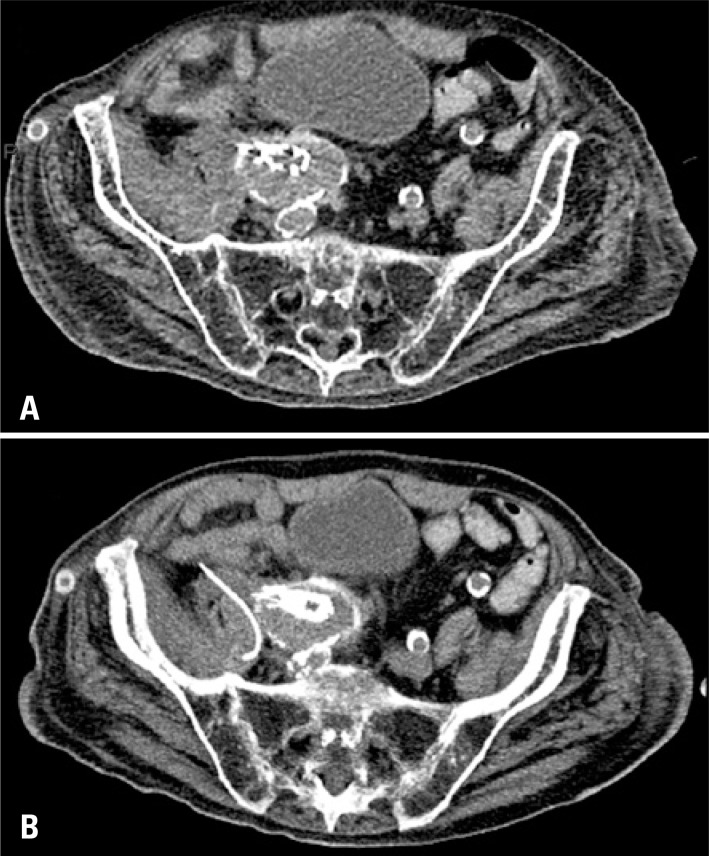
Percutaneous treatment of endograft-related psoas abscess. (A) Non-contrast CT scan confirmed the presence of fluid collection in the right psoas muscle, directly adjacent to the iliac segment of the endograft, and fat densification; (B) Computed tomography -guided percutaneous drainage with placement of a 10F pigtail catheter
